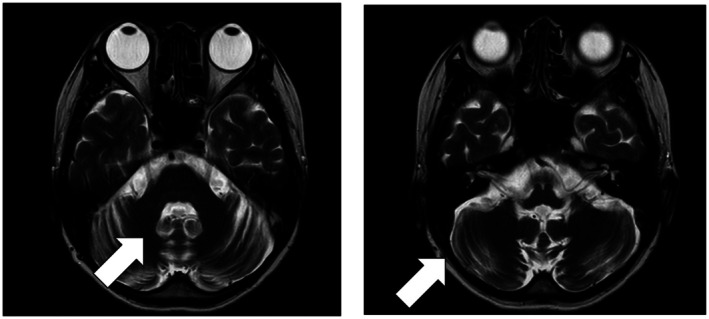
Head MRI showing atrophy of the brainstem and cerebellum.
